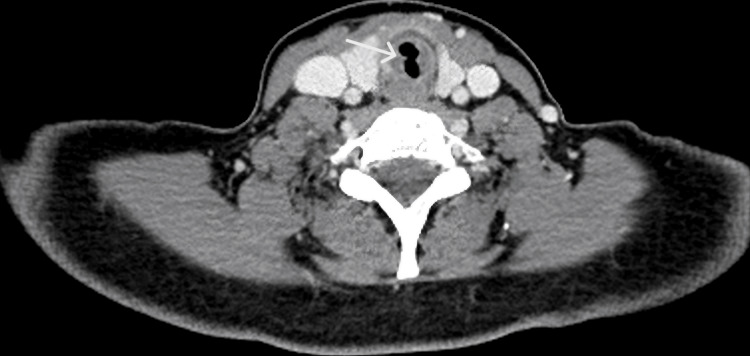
Axial CT showing upper tracheal stenosis at the narrowest point
